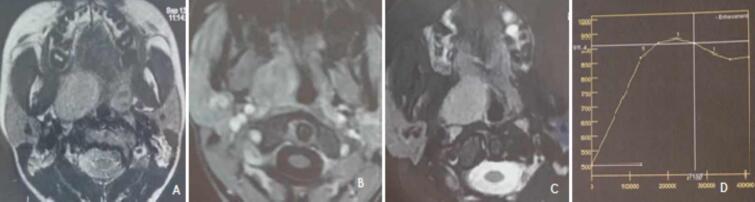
MRI of the oropharynx in axial section; A: T1 signal not injected; B: T1 signal injected; C: T2 signal; D: enhancement curve.
